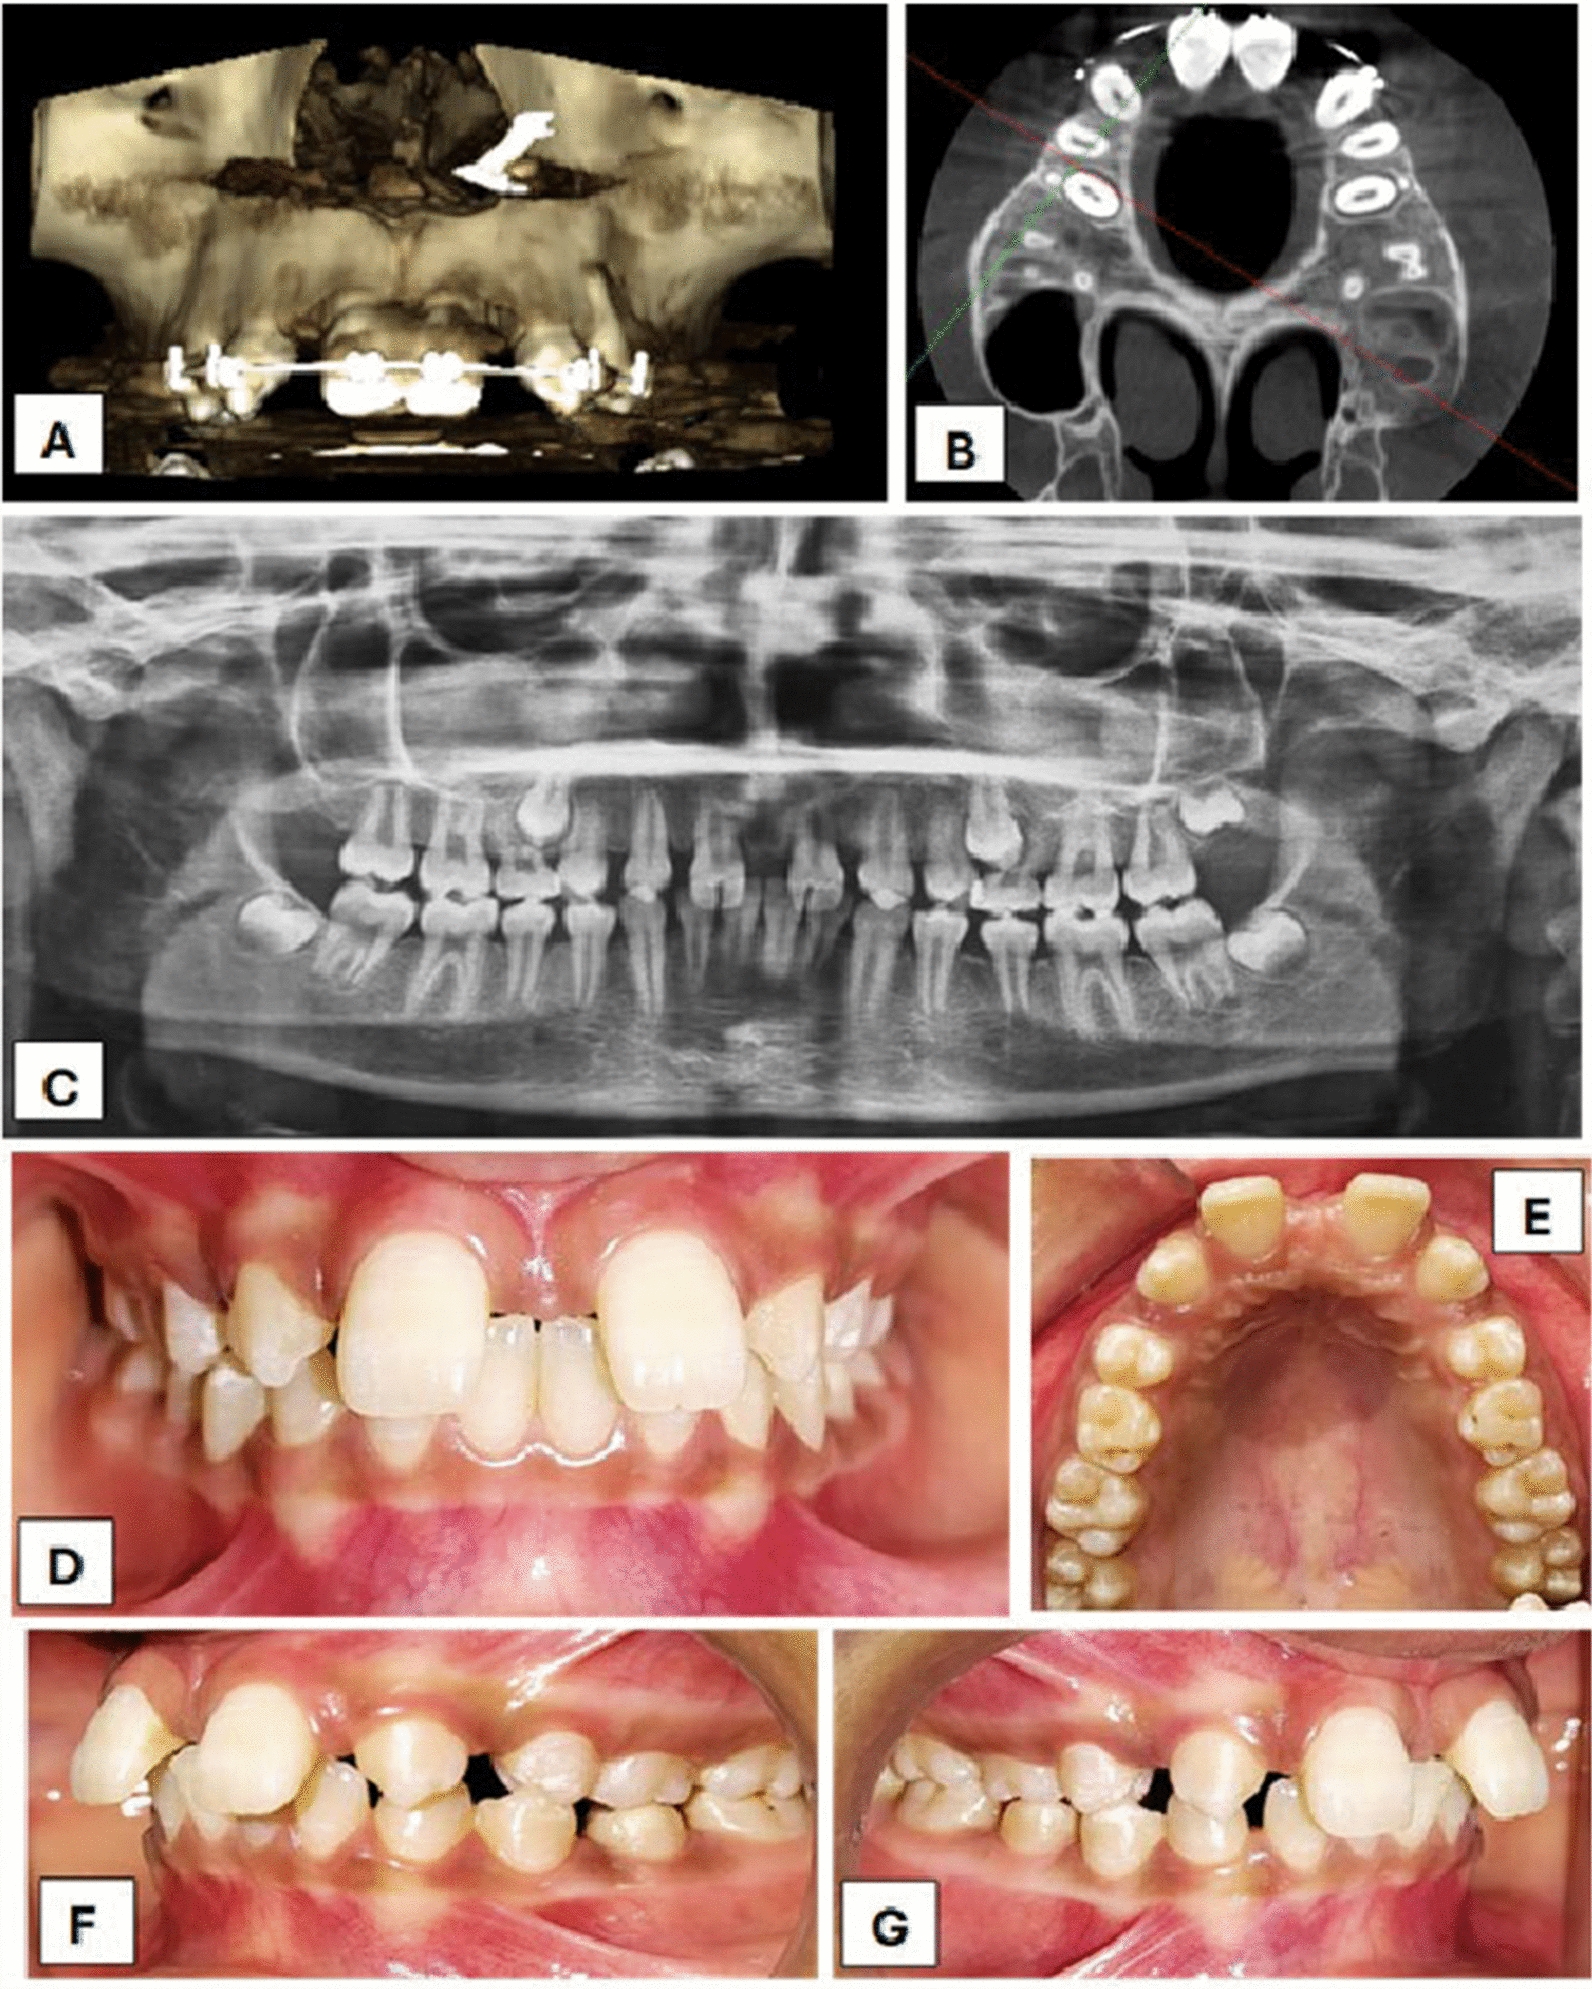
Intraoperative images A and B showing cone beam computed tomography in the mid-orthodontic phase spacing between 11 and 13, and 21 and 23. C Preoperative orthopantomograph. D Intraoral preoperative labial view. E Occlusal view. F, G Right and left buccal view
